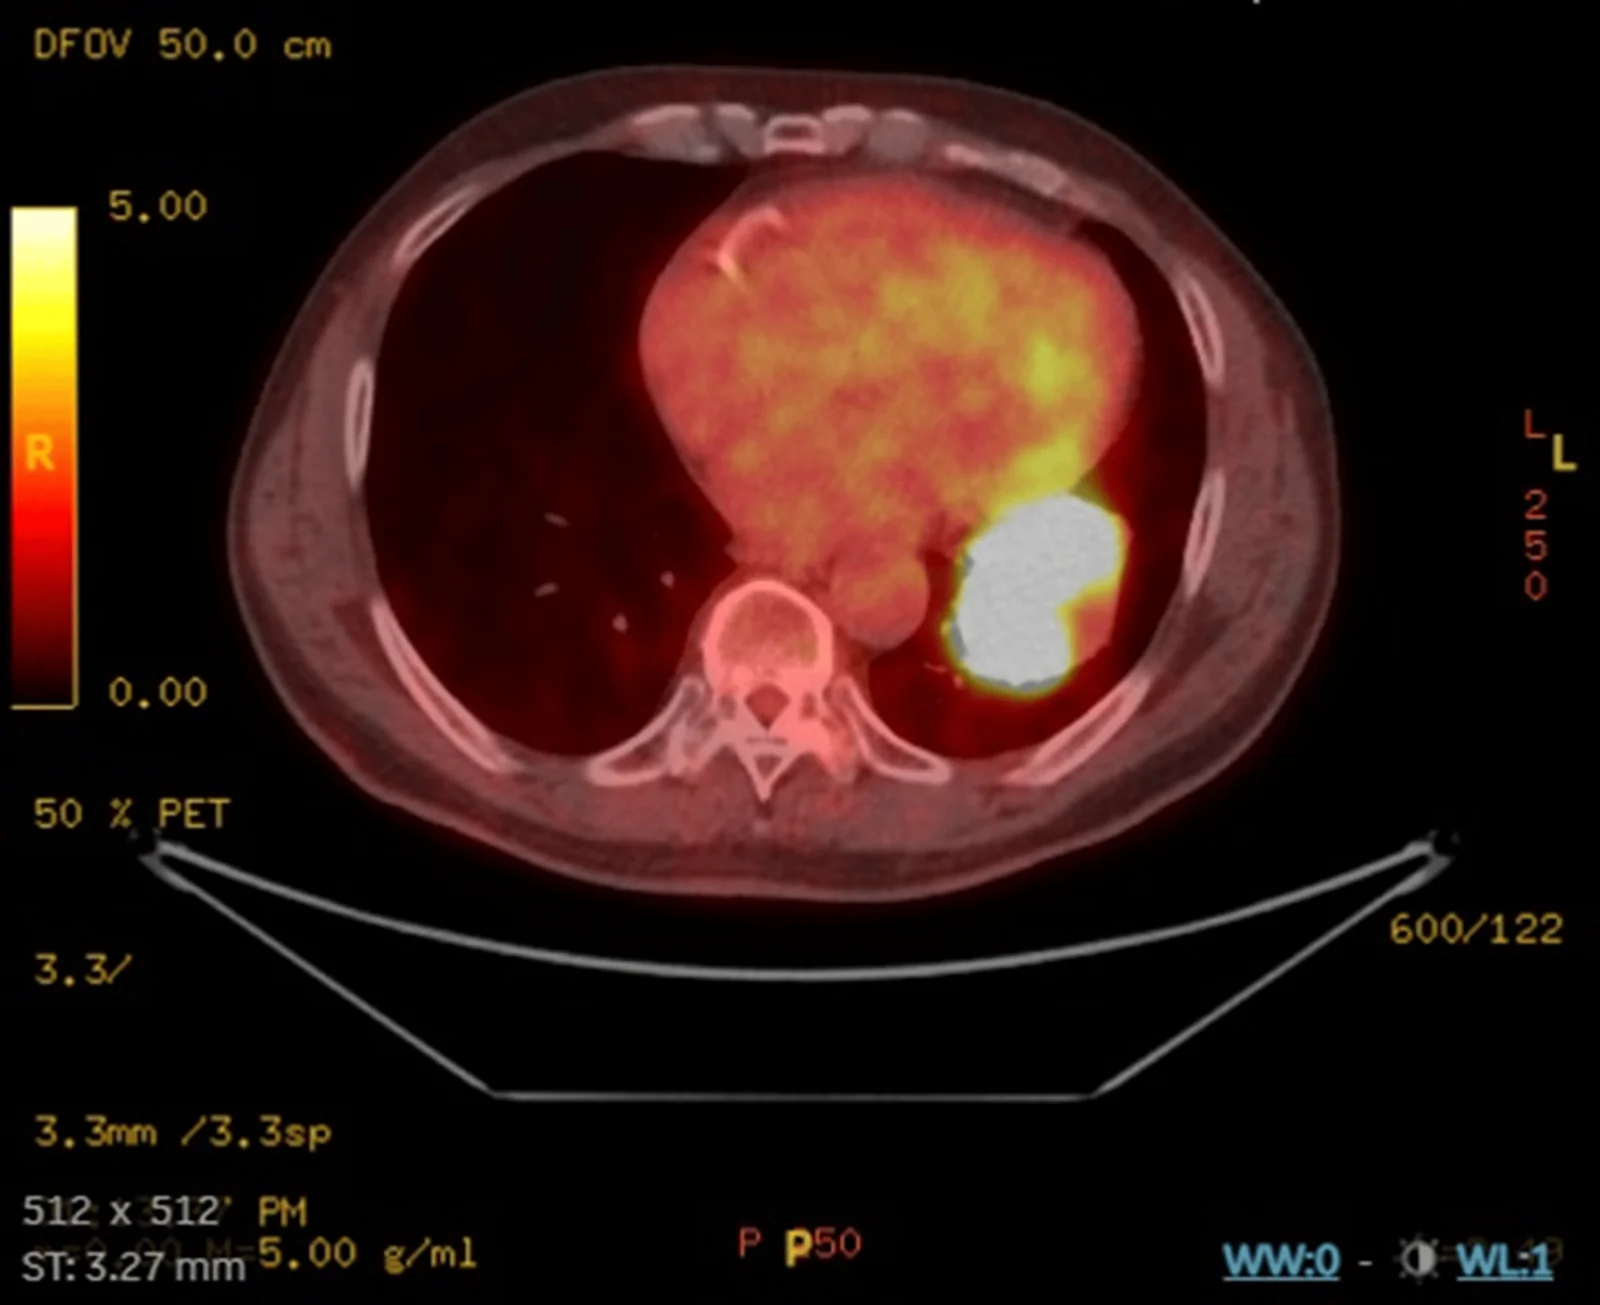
PET/CT demonstrating intense FDG uptake within the left lower lobe pulmonary mass Whole-body FDG PET/CT from the skull base to the mid-thigh demonstrates intense FDG uptake within a 5.5 cm left lower lobe pulmonary mass, consistent with hypermetabolic activity. Axial PET/CT imaging demonstrates the lesion’s relationship to the adjacent cardiac and mediastinal structures PET/CT: positron emission tomography/computed tomography; FDG: fluorodeoxyglucose
